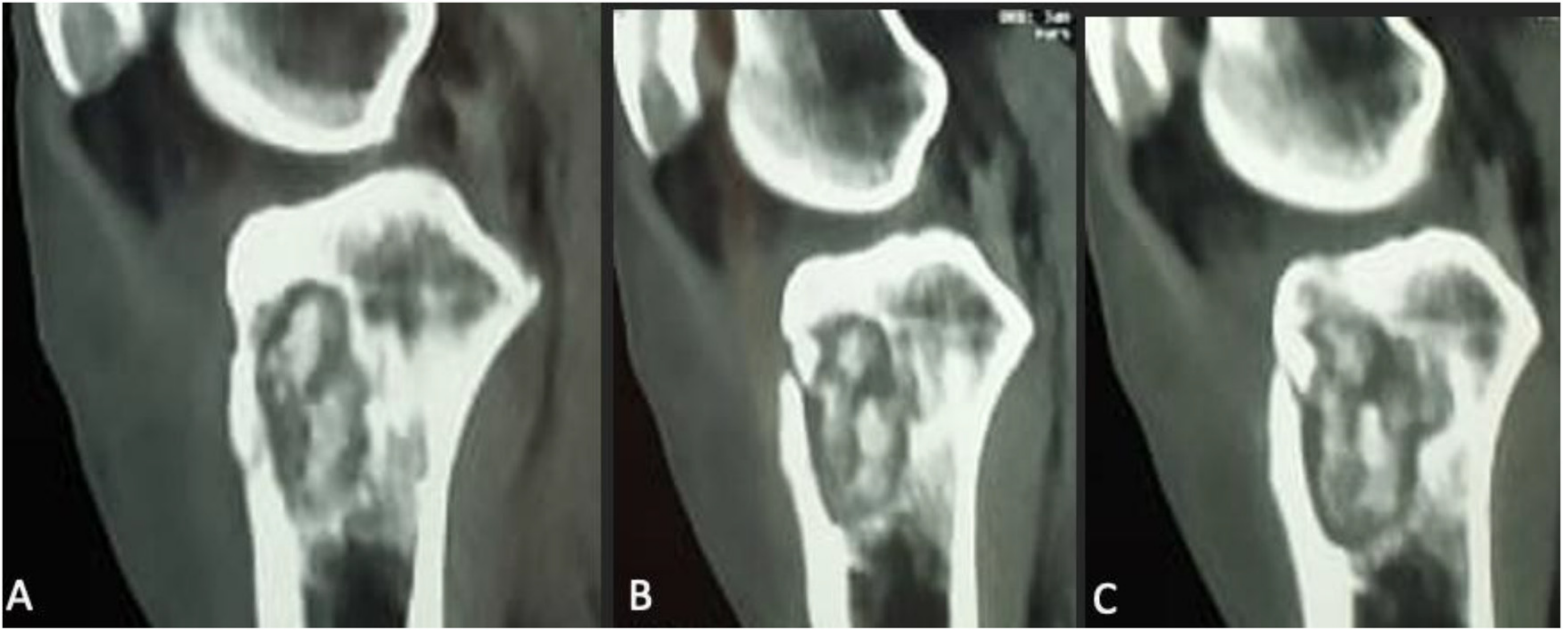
Sagittal CT scans without contrast injection of the knee show a well-defined cavitary lytic lesion (A) in the metaphyseal-epiphyseal region of the tibia, with heterogeneous content and disruption of the anterior cortex consistent with a pathologic fracture (B and C).
